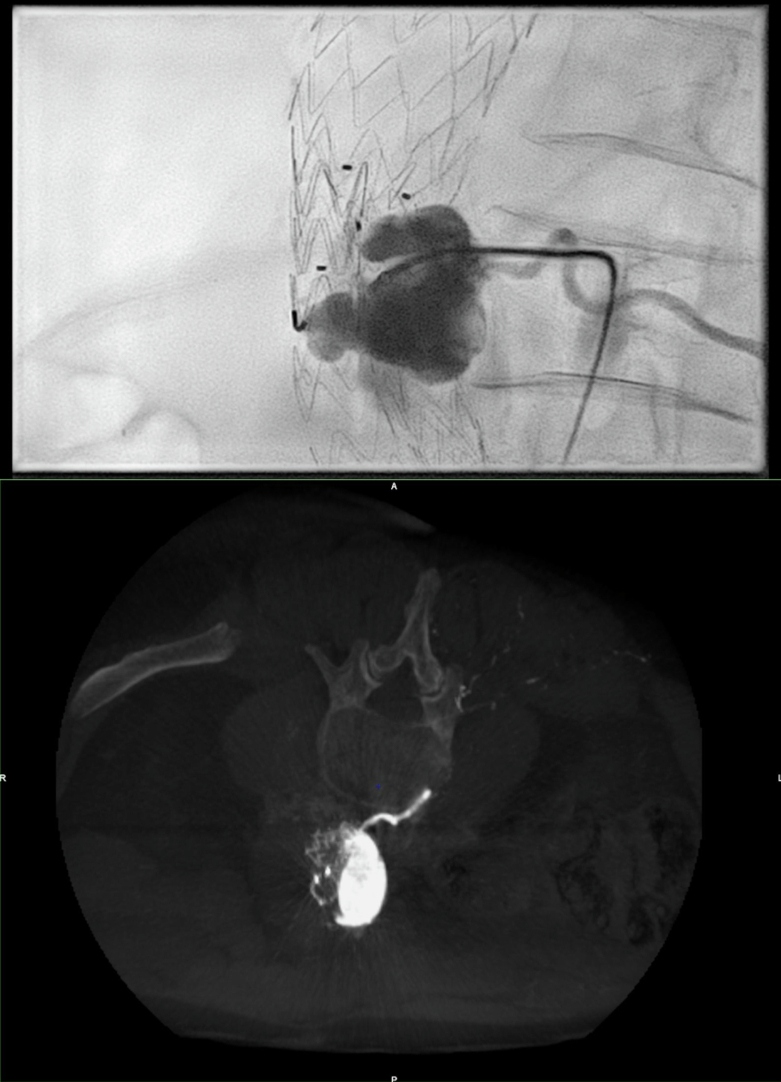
(a) Arteriography and (b) cone bean CT showing embolization of an lombar artery by aneurysmal sac direct puncture.
